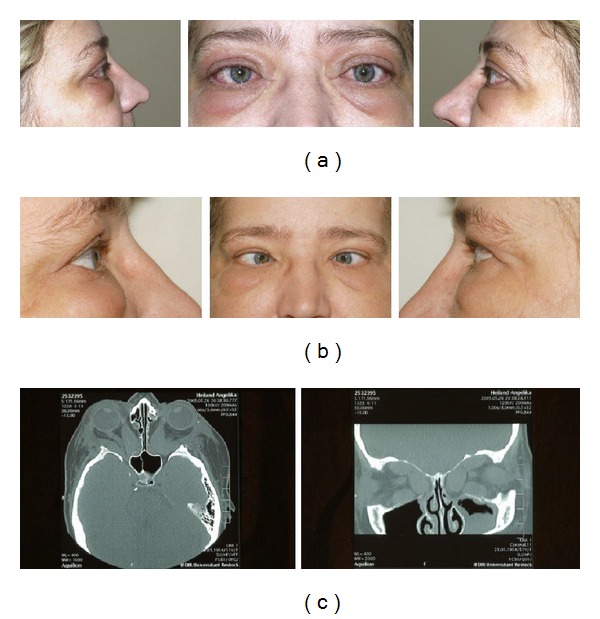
51-year-old female patient. (a) Preoperatively: severe active TAO with DON (visual acuity OD 0.6; OS 0.4), no diplopia. (b) Six months after combined medial and lateral wall decompression: visual acuity improved to OD 0.8 and OS 0.6; esotropia was induced leading to double vision in all directions of gaze. (c) Postoperatively: CT scan demonstrates definite movement of the rectus muscles into the newly created space in the paranasal sinuses and temporal fossa.
